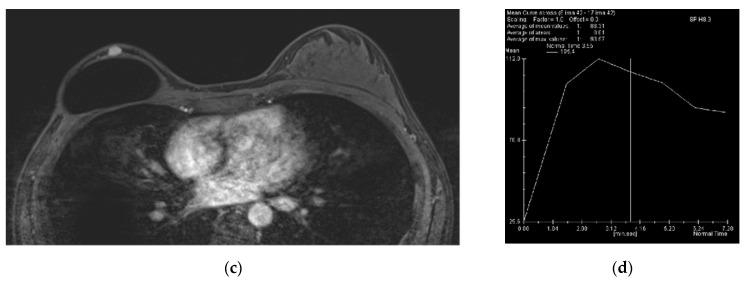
Symptomatic recurrent case mimicking a benign epidermal inclusion cyst. A 27-year-old female patient who underwent right-breast skin-sparing mastectomy with reconstruction with a silicone implant bag for mucinous carcinoma (grade 2, luminal type) 48 months ago. (a,b) Using ultrasound as a method of routine surveillance, an oval circumscribed hypoechoic mass with posterior enhancement was noted in the reconstructed breast, where the patient complained that a palpable lump was present. Since the mass is accompanied by skin tract and showed no vascularity on the Doppler scan, it was considered to be an epidermal inclusion cyst. (c,d) At postoperative MRI, the mass showed fast enhancement on the early dynamic phase fat-saturated T1-weighted image with a washout kinetic pattern. Based on the finding of the MRI scan, the category of the mass was upgraded to category 4, and it was confirmed as invasive ductal carcinoma by surgical excision.
